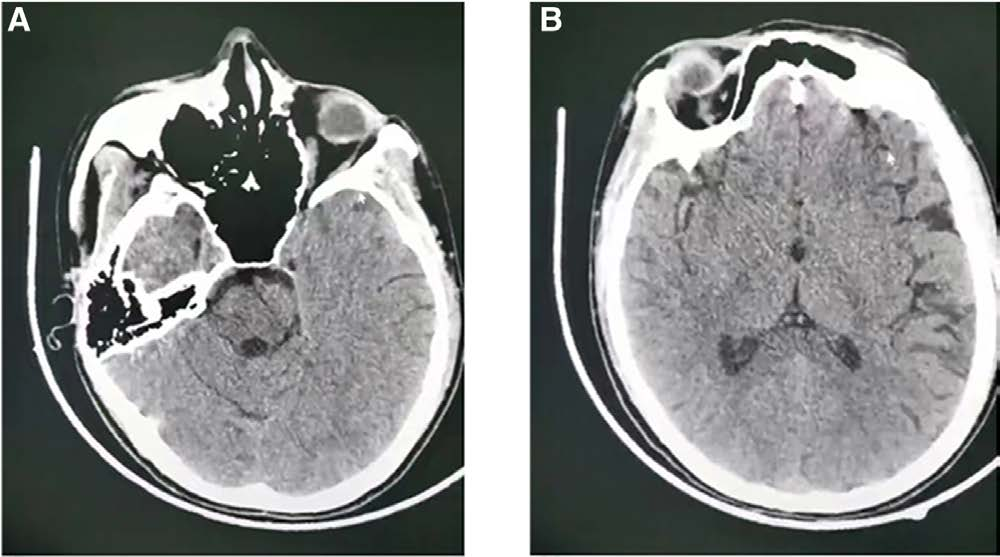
Brain computed tomography imaging of the patient at onset. (A, B) No definite hemorrhagic foci and large infarcts in brain parenchyma were found.
